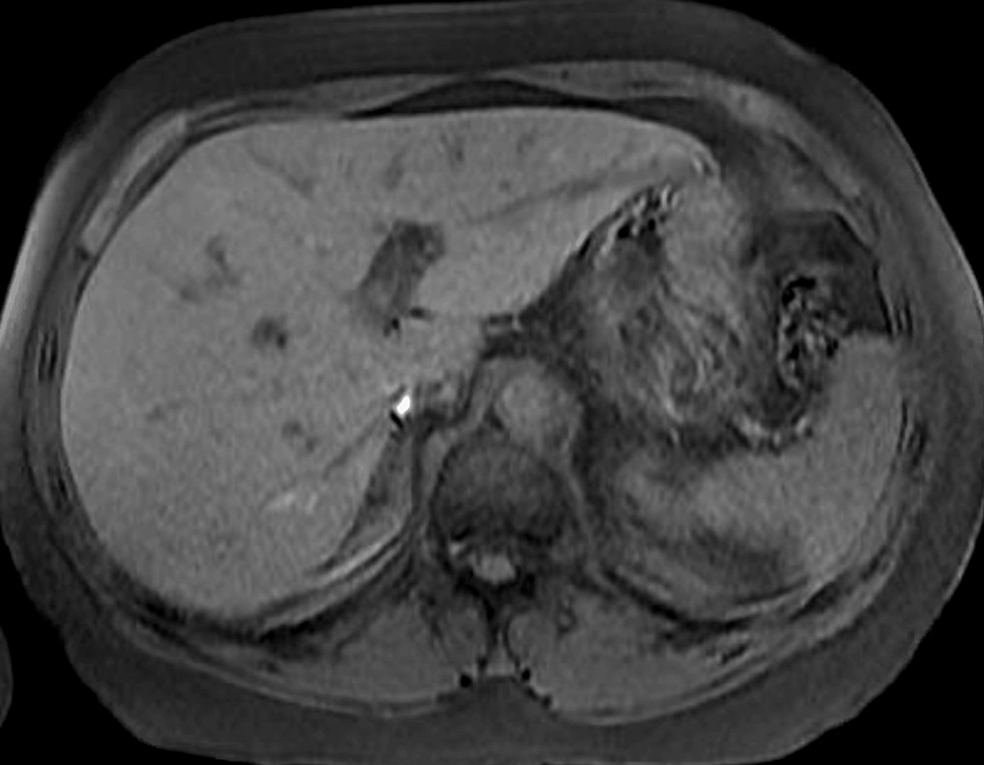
Hospital day 2: MRI MRCP T1 phase MRCP: magnetic resonance cholangiopancreatography
